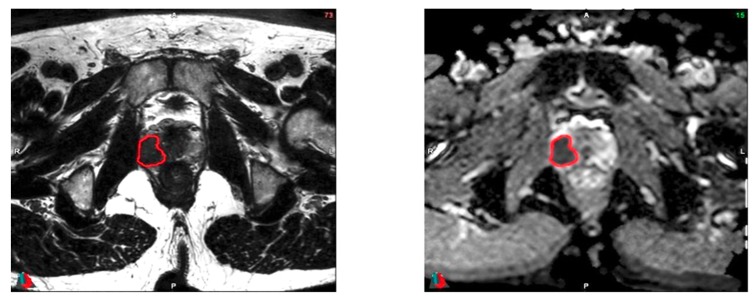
Examples of delineation on both T2 (left) and ADC (right) sequences. Images acquired on a Philips 3T Scan. Patient #27: Initial PSA (Prostate Specific Antigen) of 6.80 ng/mL, no clinical anomaly. MRI: suspicion of extracapsular extension. Pathology exam: Gleason score of 9 (4 + 5), bilateral invasion of the prostate (pT2c). Magnification scale: ×1.
